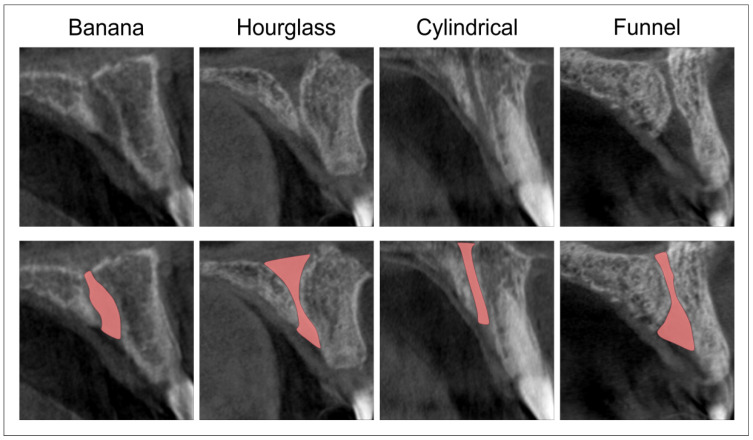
The NPC types and marks of interest. Upper: Sagittal CBCT cross-sections; bottom: red marks define NPC types.
